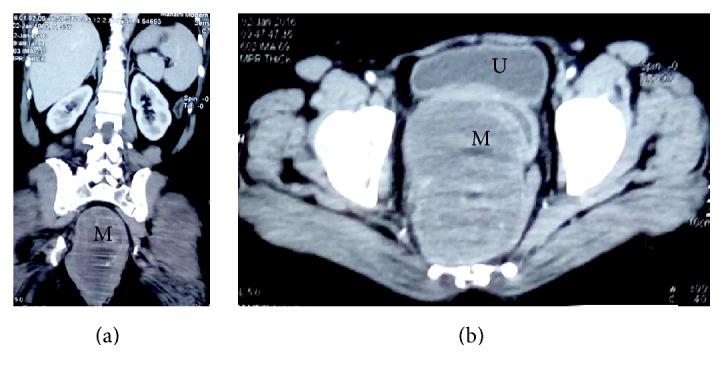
Contrast enhanced computed tomography (CT). (a) Frontal section showing the extension of the mass (M) from the sacrum to the perineum. (b) Transverse section showing the mass (M) compressing the bladder (U) and vaginal canal anteriorly and the rectum posteriorly.
